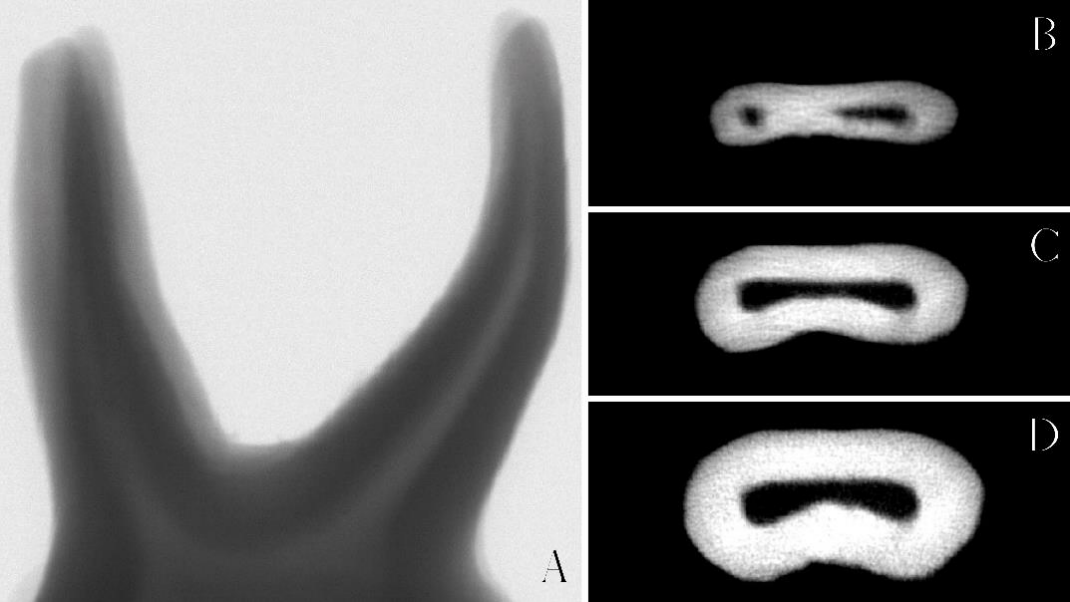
Microcomputed tomography scanned full image of primary molar (A) demonstrating three-dimensional reconstruction and the superimposed of the 3D thirds slices reconstruction, used for the measurements analyses according to the root thirds: apical (B), middle (C), and cervical (D).
